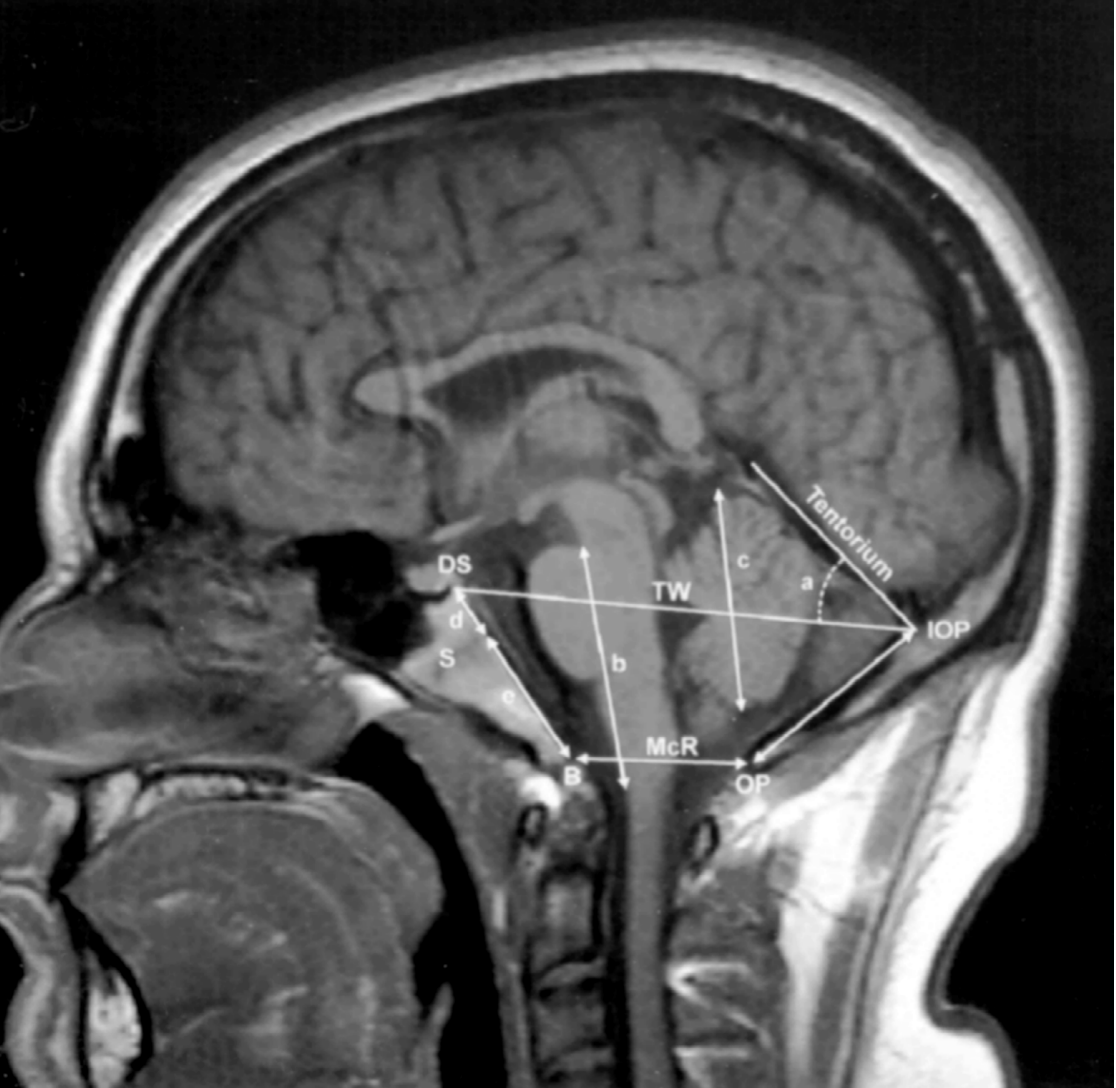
A T_1_-weighted sagittal MR image from a control subject, showing the midline structures of the posterior cranial fossa and the brainstem and the cerebellum. d + e = length of clivus; S = sphenooccipital synchondrosis; d = length of basisphenoid between the top of the dorsum sellae and the sphenooccipital synchondrosis of the clivus; e = length of the basiocciput between the synchondrosis and the basion; b = length of the hindbrain between the midbrain-pons junction and the medullocervical junction; a = angle of the cerebellar tentorium to Twining's line; c = length of cerebellar hemisphere; DS = top of the dorsum sellae; IOP = internal occipital protuberance; OP = opisthion; IOP to OP = length of supraocciput; B = basion; TW = Twining's line; McR (B to OP) = McRae's line.
